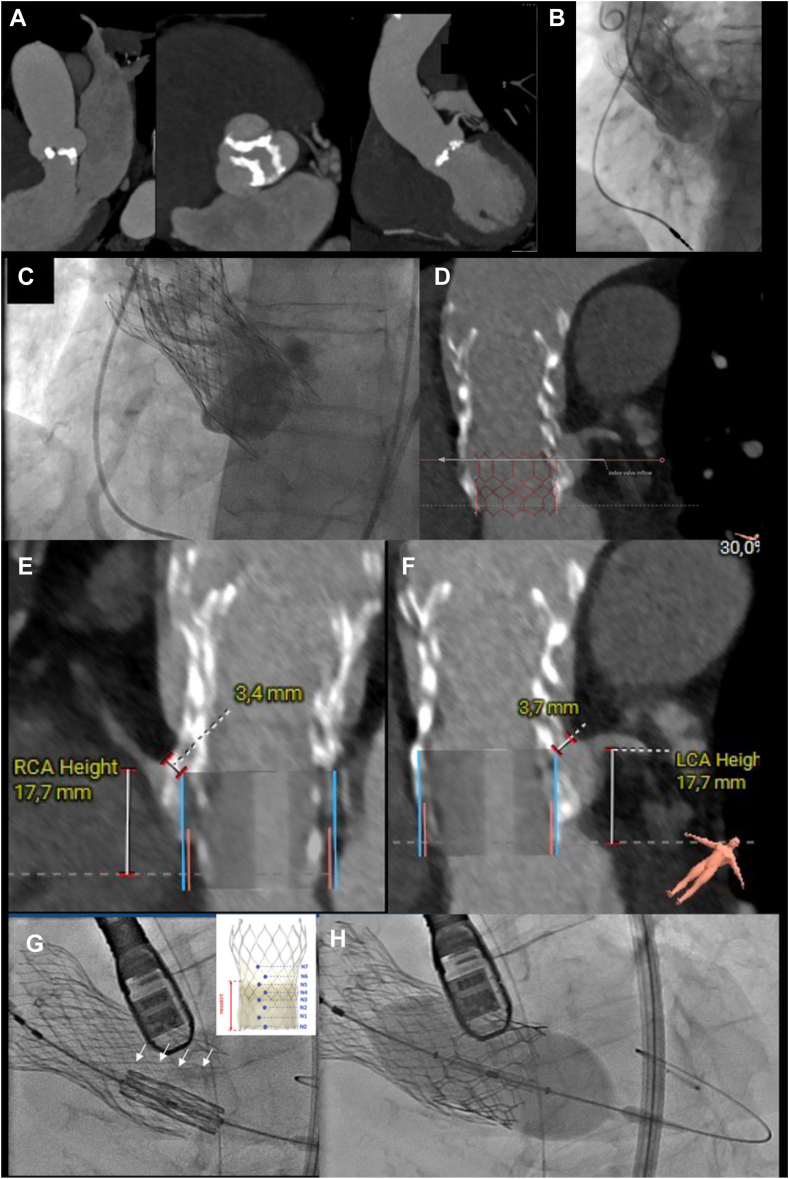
(A) Computed tomography scan pre-initial transcatheter aortic valve (TAV) implantation showing bicuspid anatomy. (B) Implantation of the first transcatheter heart valve (too high) with a significant aortic regurgitation. (C) Final result of TAV in series. (D-F) Computed tomography of pre TAV-in-TAV-in TAV. (G) Image before Edwards SAPIEN-3 Ultra (Edwards Lifesciences, Irvine, CA) valve deployment at node 4. (H) Postdilatation of the transcatheter heart valve. LCA, left coronary artery; RCA, right coronary artery.
